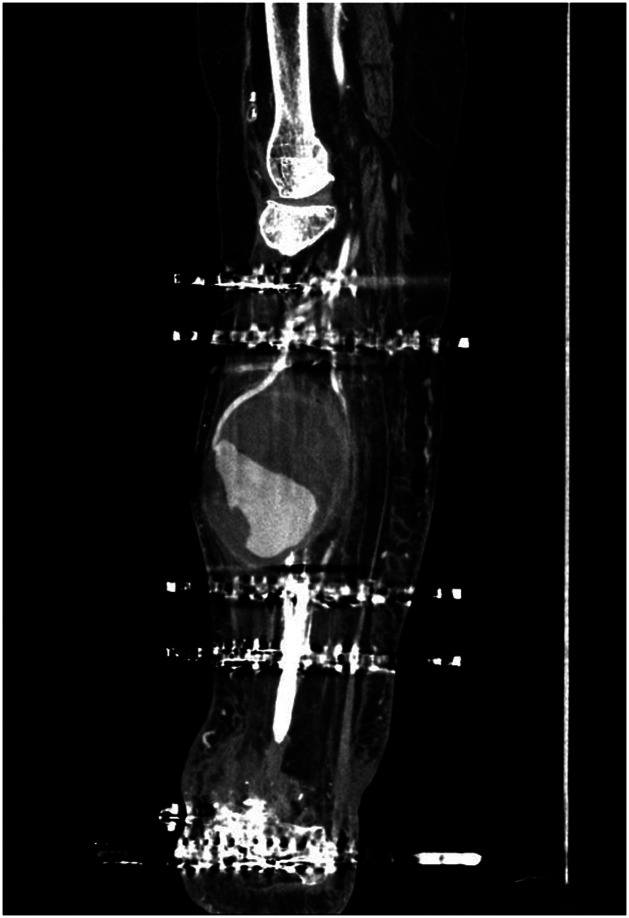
Sagittal computed tomography angiography-based MPR reconstruction showing a partially thrombosed pseudoaneurysm (hypodense upper portion) with a fine neck communicating it to the anterior tibial artery.
